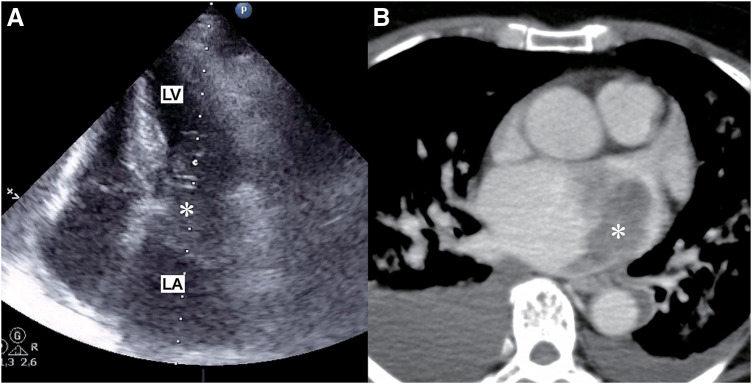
Echocardiography and CT scans. The echocardiogram (A) and contrast-enhanced CT radiograph (B) acquired upon the initial arrival of the patient show that a large tumor (asterisk) in the left atrium was incarcerated in the mitral annulus. CT, computed tomography; LA, left atrium; LV, left ventricle
